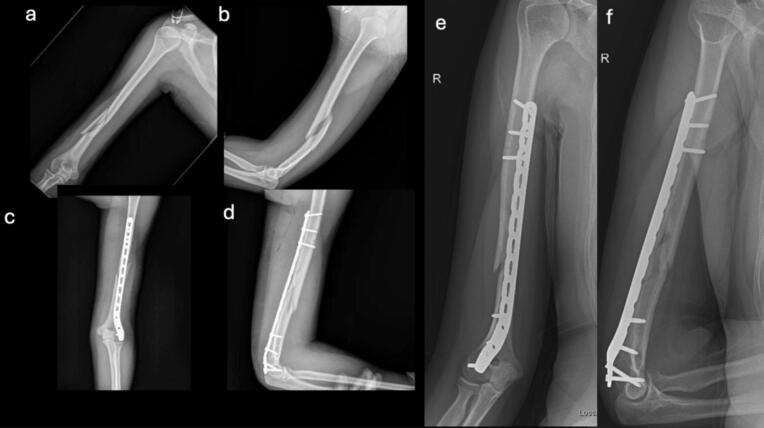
Case 1 – Female patient, 35 years old, mechanism of injury: fall from standing height. a-b) Preoperative AP and lateral radiographs showing AO 1.2.B.2-c fracture; c-d) Immediate postoperative AP and lateral radiographs; e-f) AP and lateral radiographs at 1-month follow-up.
